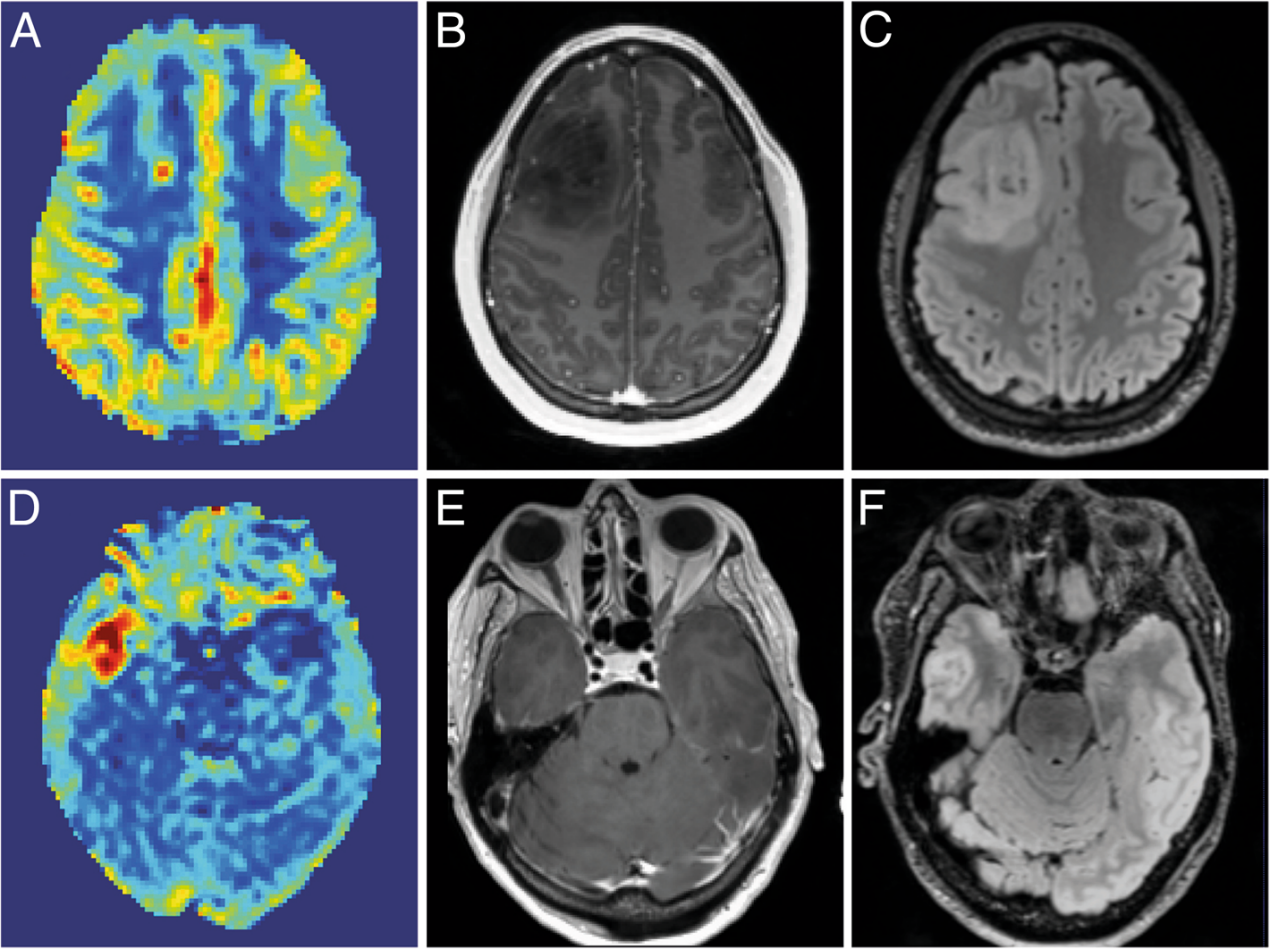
a–c Example of a 24-year-old female patient, with oligodendroglioma (grade II) in the left frontal lobe. a Cerebral blood flow, imaged by ASL. Red indicates high blood flow. b T1-Gd and c FLAIR. d–f Example of a 63-year-old female patient with GBM (grade IV) in the right temporal lobe. d Cerebral blood flow, imaged by ASL. Red indicates high blood flow. e T1 post Gd. f FLAIR. A clear difference in tumour blood flow can be seen between both patients (courtesy: A. Alsaedi, S. Bisdas)
